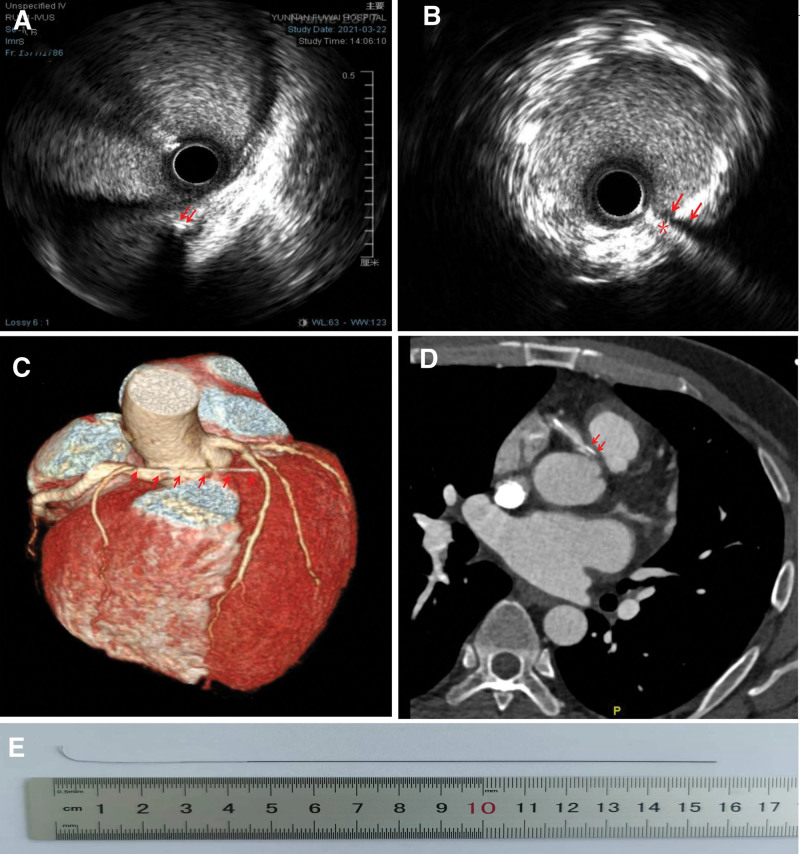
(A) The IVUS revealed that the fractured guidewire had penetrated the adventitia of the proximal RCA (red arrow). (B) The mid-segment of the guidewire was lodged by the previously implanted stent (red arrow). (C) CTA, axial view. The proximal end of the guidewire had already perforated the RCA wall (red arrow). (D) The CTA showed that the guidewire had penetrated the anterior wall of the left ventricle (red arrow). (E) The 16-cm broken BMW wire fragment was removed surgically. IVUS = intravascular ultrasound; RCA = right coronary artery.
